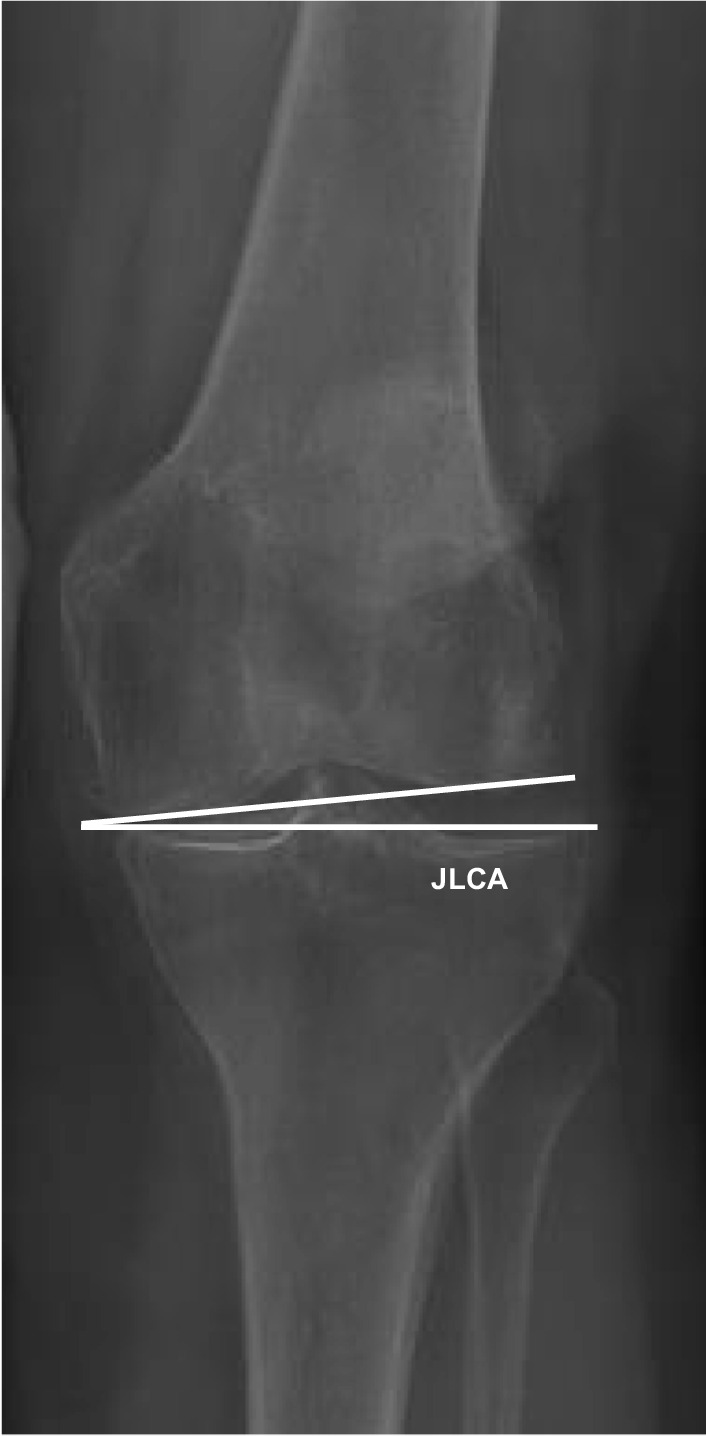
Joint line convergence angle (JLCA). Close-up of the knee joint of a pre-operative, weightbearing lower-limb anteroposterior EOS digital radiograph is shown. The JLCA is the angle formed by the articular surfaces of the femur and tibia
